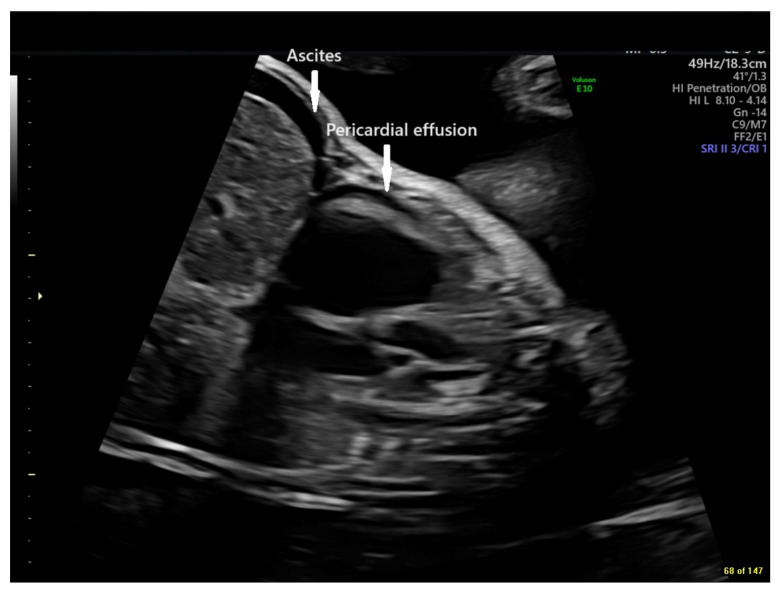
Echocardiographic sagittal still frame image of the fetal chest and abdomen demonstrating ascites and pericardial effusion (arrows) in the setting of early fetal hydrops at 30 6/7 weeks gestation (GE Voluson E10 Imaging System).
